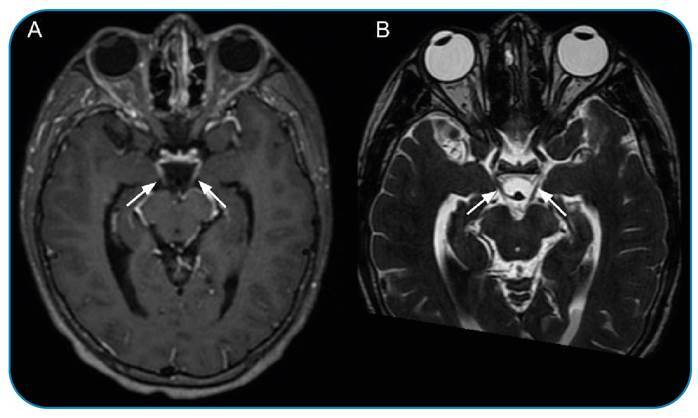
Magnetic resonance images of the brain show bilateral enhancement and thickening of the cisternal segments of the oculomotor nerves at T1-weighted contrast-enhanced (A) and high-resolution T2-weighted (B) sequences. Lateral deviation of the right eyeball can be observed.
